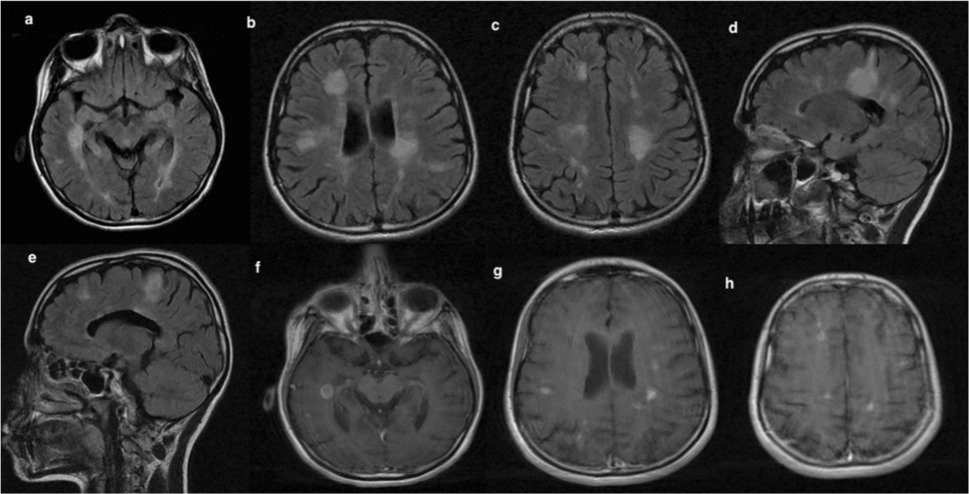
Follow-up MRI brain scans (March 2014) showed a significant increase in the lesion load involving the periaqueductal gray matter (a), periventricular and juxtacortical regions (b,c), corpus callosum, extending lesions to cortical areas (d,e) in both axial and sagittal T2/flair scans. Enhanced axial T1 MRI scans showed the presence of new gadolinium-enhancing lesions in the temporal, frontal and parietal lobes (f-h).
